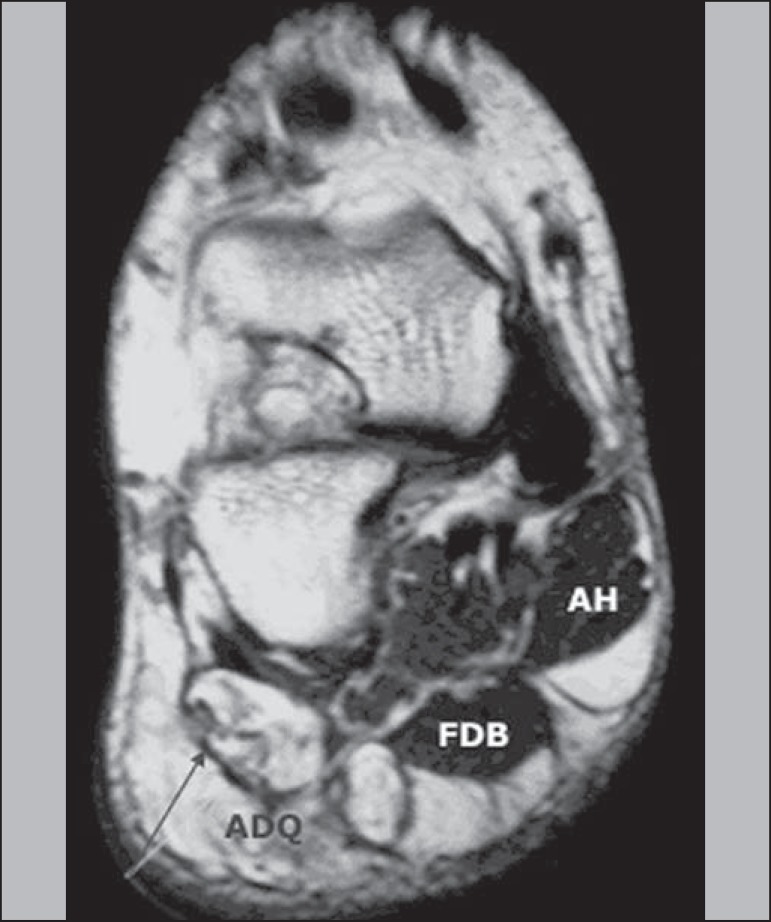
Coronal MRI T2-weighted image without fat saturation showing significant
